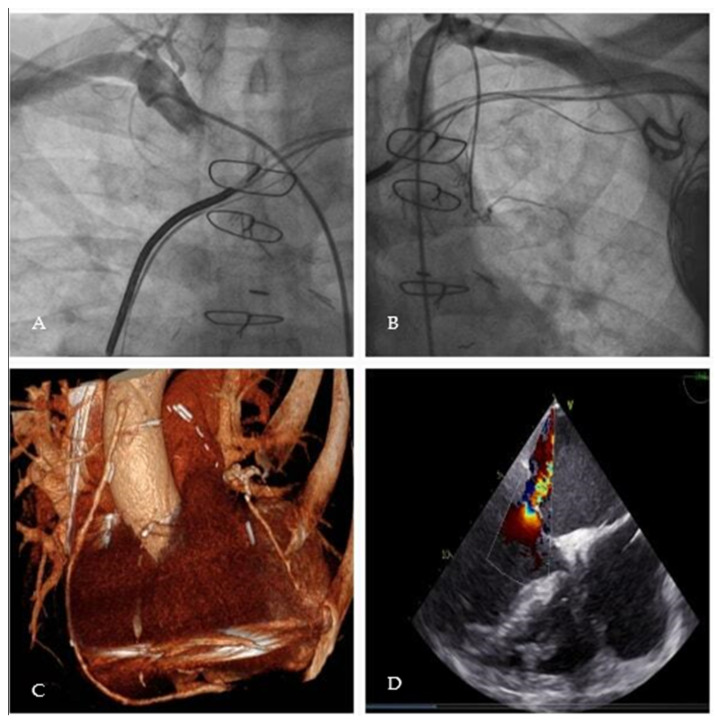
(A): RIMA occluded and (B): LIMA occluded at coronary angiography; (C): SVG to PDA, only patent graft at chest CT scan; (D): pre-operative TEE shows a regurgitant jet that reaches the roof of the left atrium (Coanda effect).
